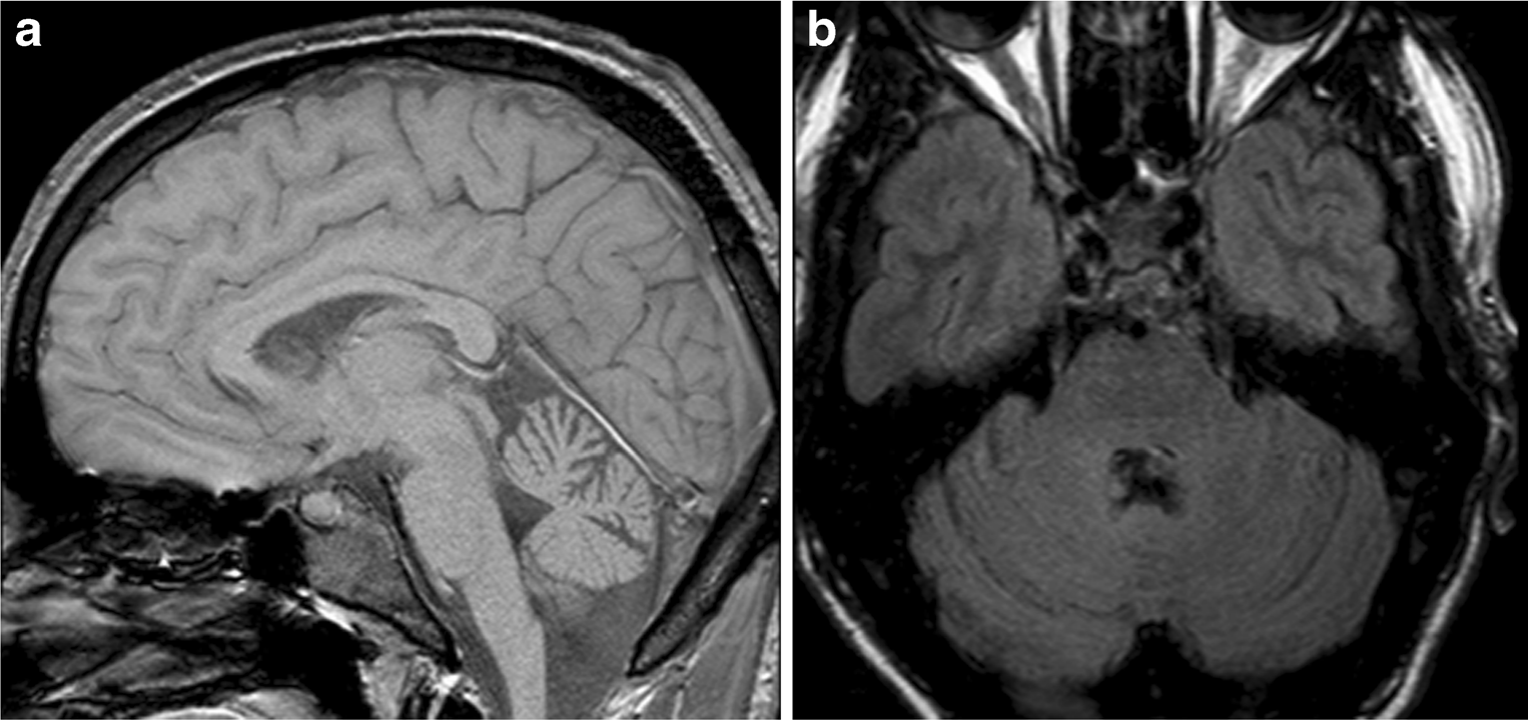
Brain MRI of patient V:1. a Midsagittal and coronal T1-weighted image. b Mild cerebellar atrophy
